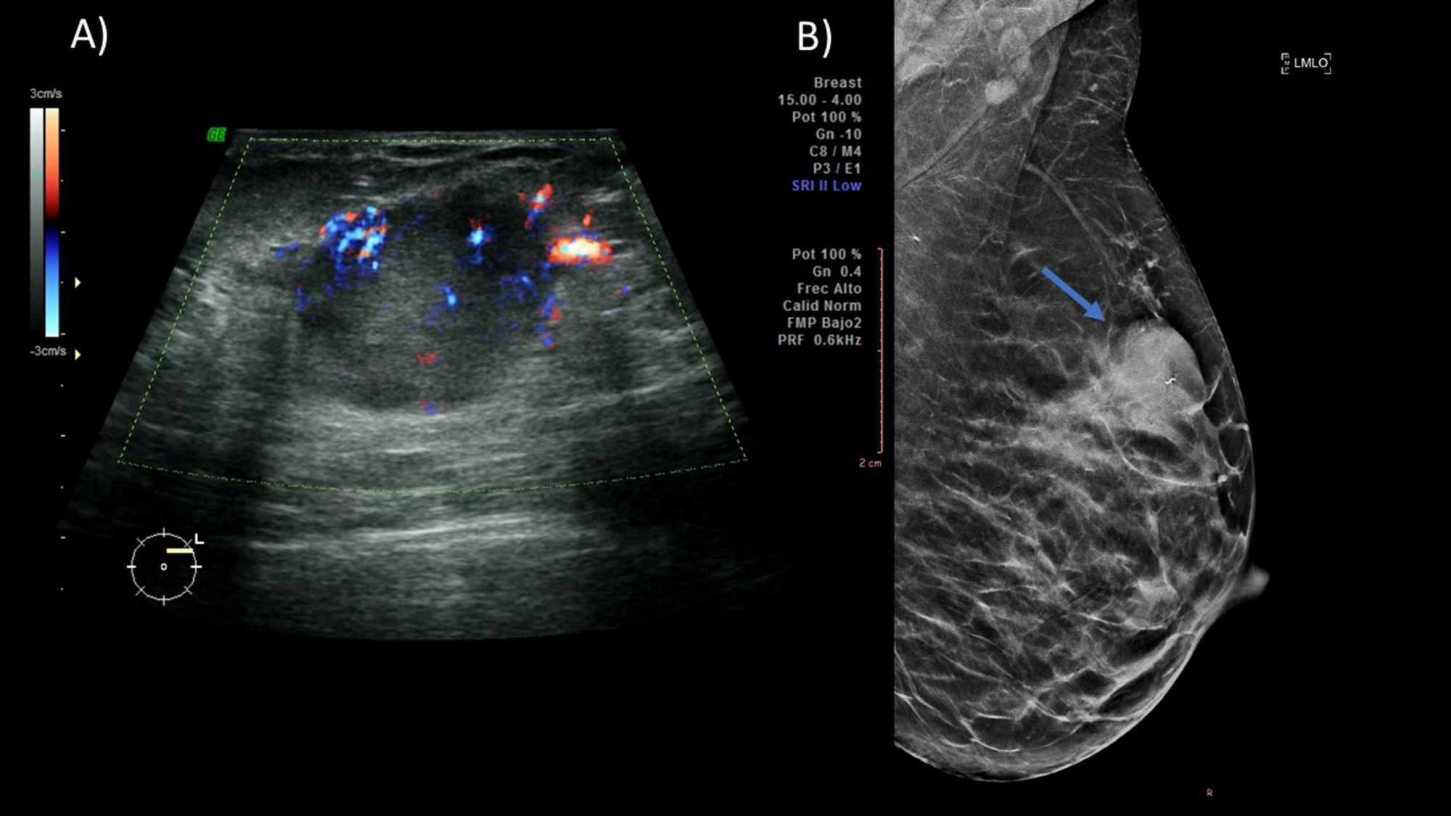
A 36-year-old female patient in her second trimester with a palpable mass in the left breast that presents growth in the last three months. (A) Ultrasound showing at 2:00 a hypoechoic nodule with micronodulated margins of 2.8 cm, and Doppler showing peripheral vascularity. (B) MLO projection of the left breast showing a hyperdense nodule of irregular margins (blue arrow) that causes skin retraction. A percutaneous biopsy was performed, with a triple-negative high-grade infiltrating ductal carcinoma result. MLO, mediolateral oblique
